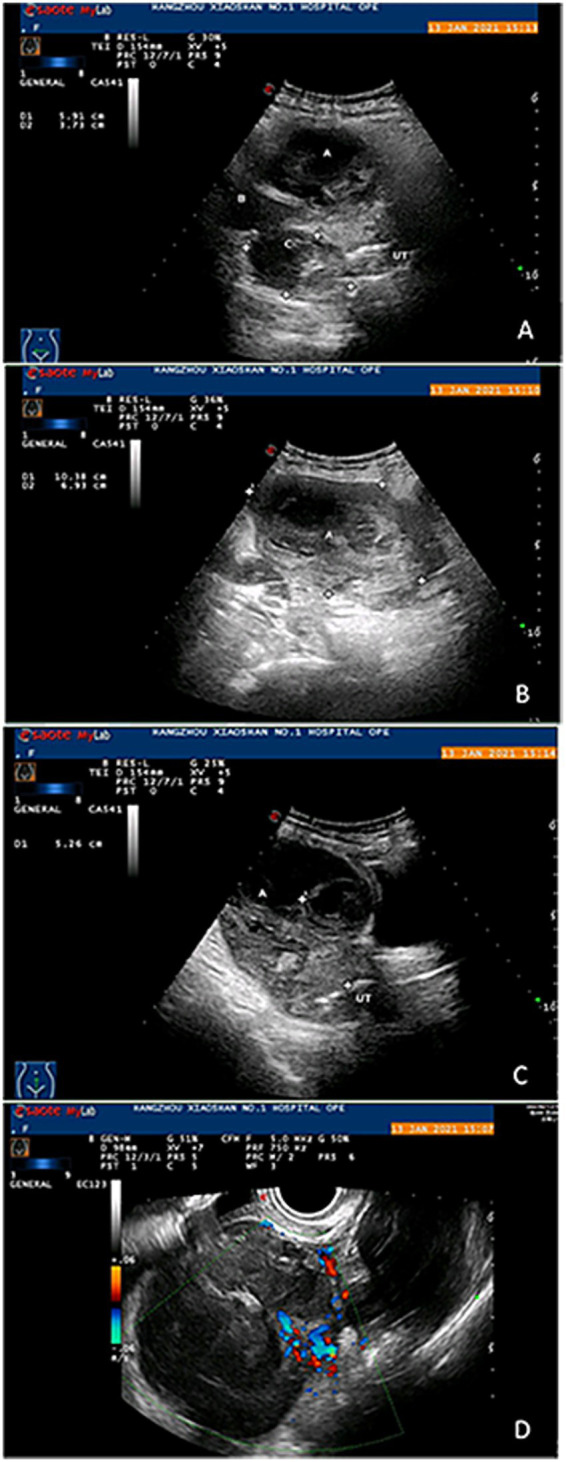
(A) Transvaginal ultrasound showing multiple solid uterine masses (marked A–C); (B) Mass A measuring 10.5 × 8.2 × 8.1 cm; (C) Intrauterine device in the uterine cavity with an irregular liquefaction area in mass A; (D) Tortuous strip blood flow signals and Adler grade II blood flow in partial tumor areas.
